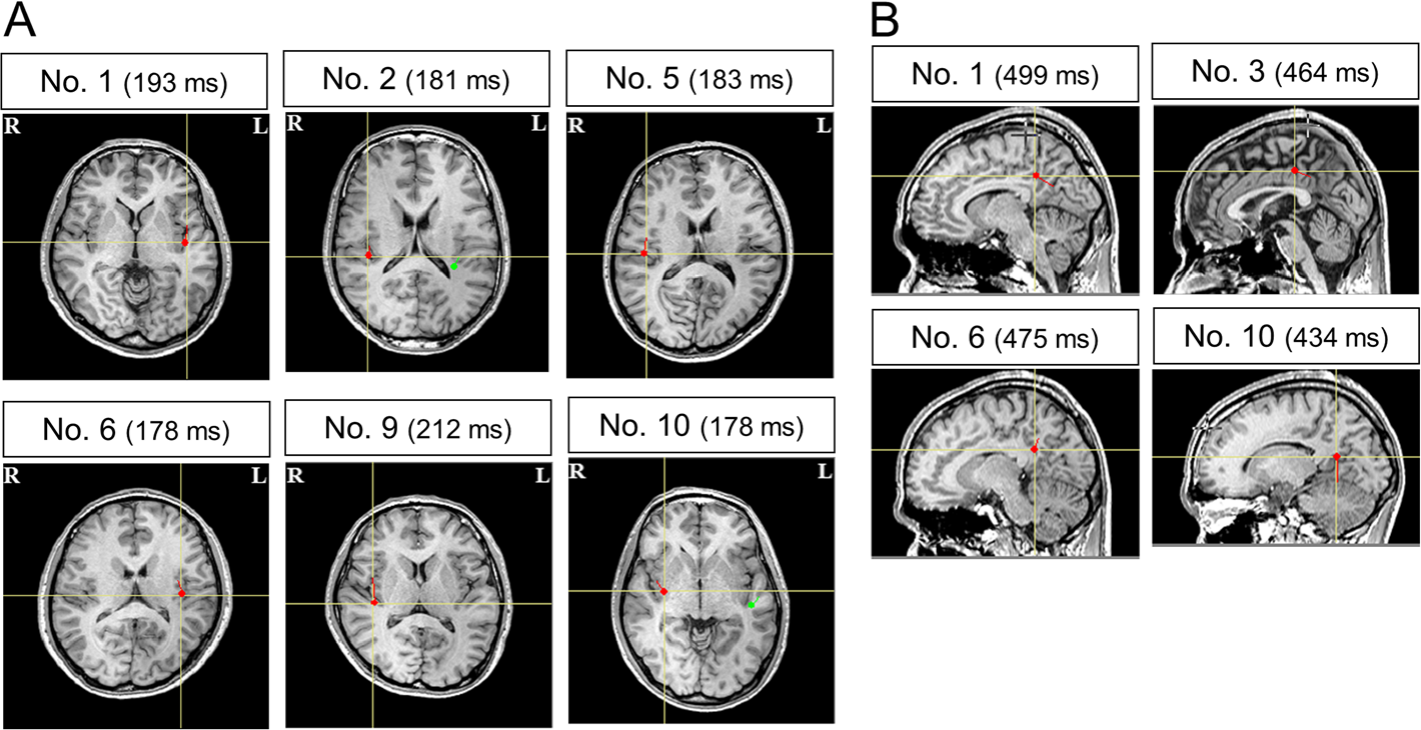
Locations of the equivalent current dipoles (ECDs) induced by the conditioned stimuli. (A) Magnetic responses with latencies approximately 190 ms were localized in the insular cingulate cortex in six participants (axial view) (R: right side; L: left side). Bilateral activations of the insular cortex were observed in participants No. 2 and No. 10. (B) Magnetic responses with latencies approximately 470 ms were localized in the posterior cingulate cortex in four participants (sagittal view). Closed red and green circles indicate the location of ECDs and short red and green lines radiating from closed circles indicate the orientation of ECDs. ECDs are superimposed on individual magnetic resonance images. The peak latencies are presented in parentheses.
